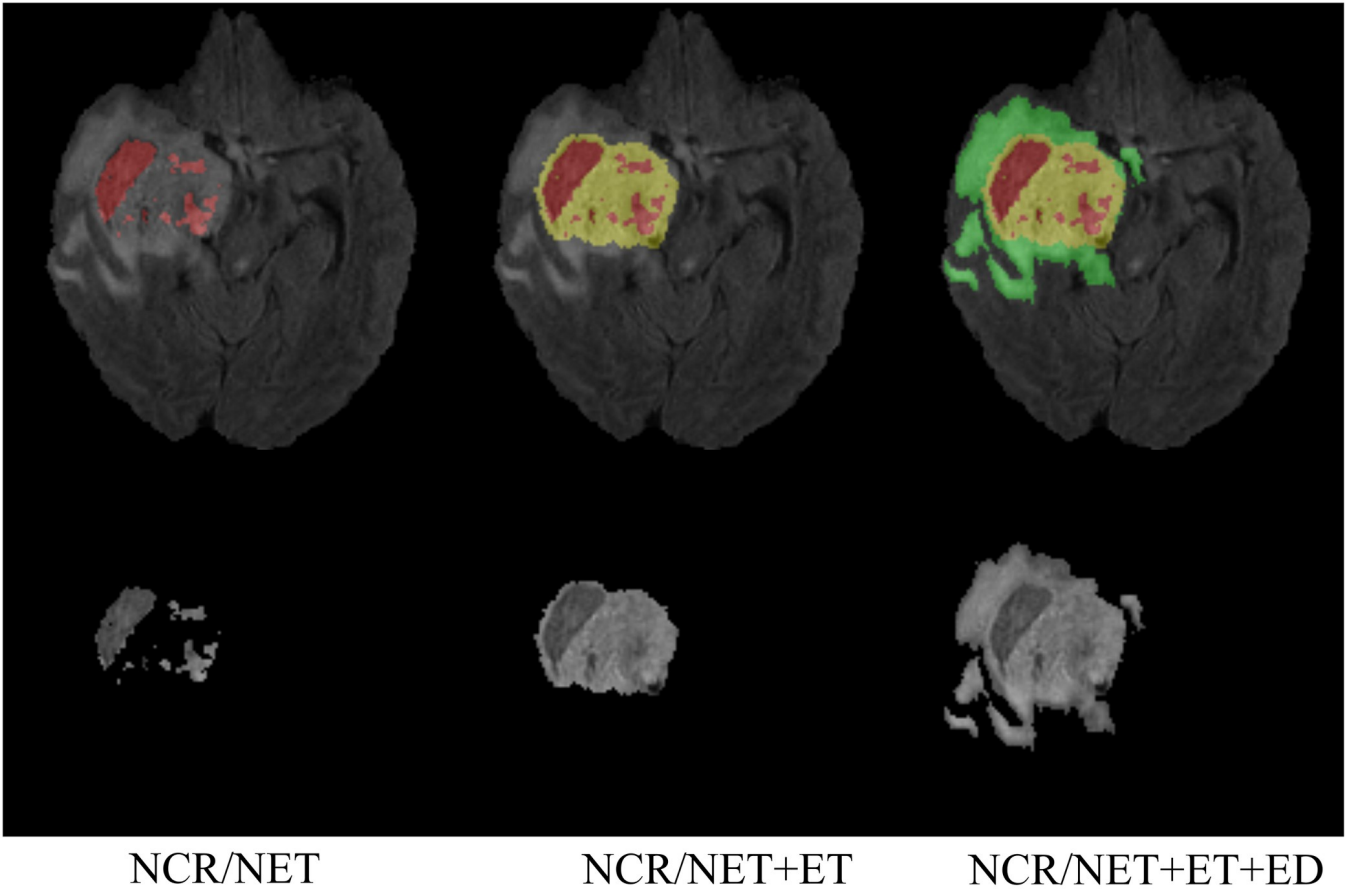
Segmentation labels for MRI images of brain gliomas. The first column composed of NCR/NET(red); the second column composed of NCR/NET(red) and ET(yellow); the third column composed of NCR/NET(red), ET(yellow) and ED(green).
